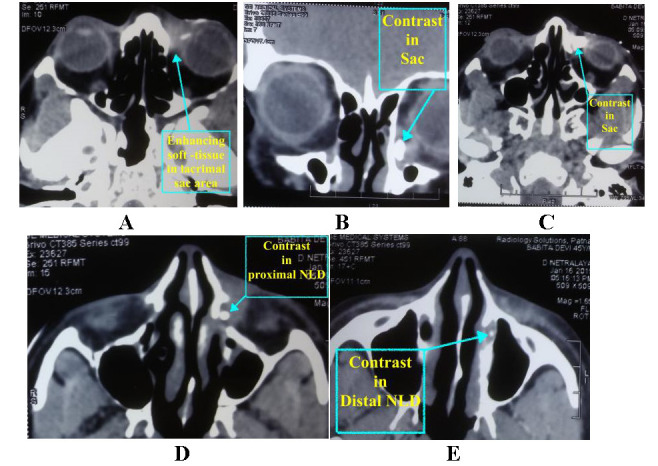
A-E Ill-defined soft tissue enhancement in the left lacrimal sac region on CECT (A); Contrast seen in the left lacrimal sac (B, C), proximal (D), and distal NLD (E) on CT-DCG
